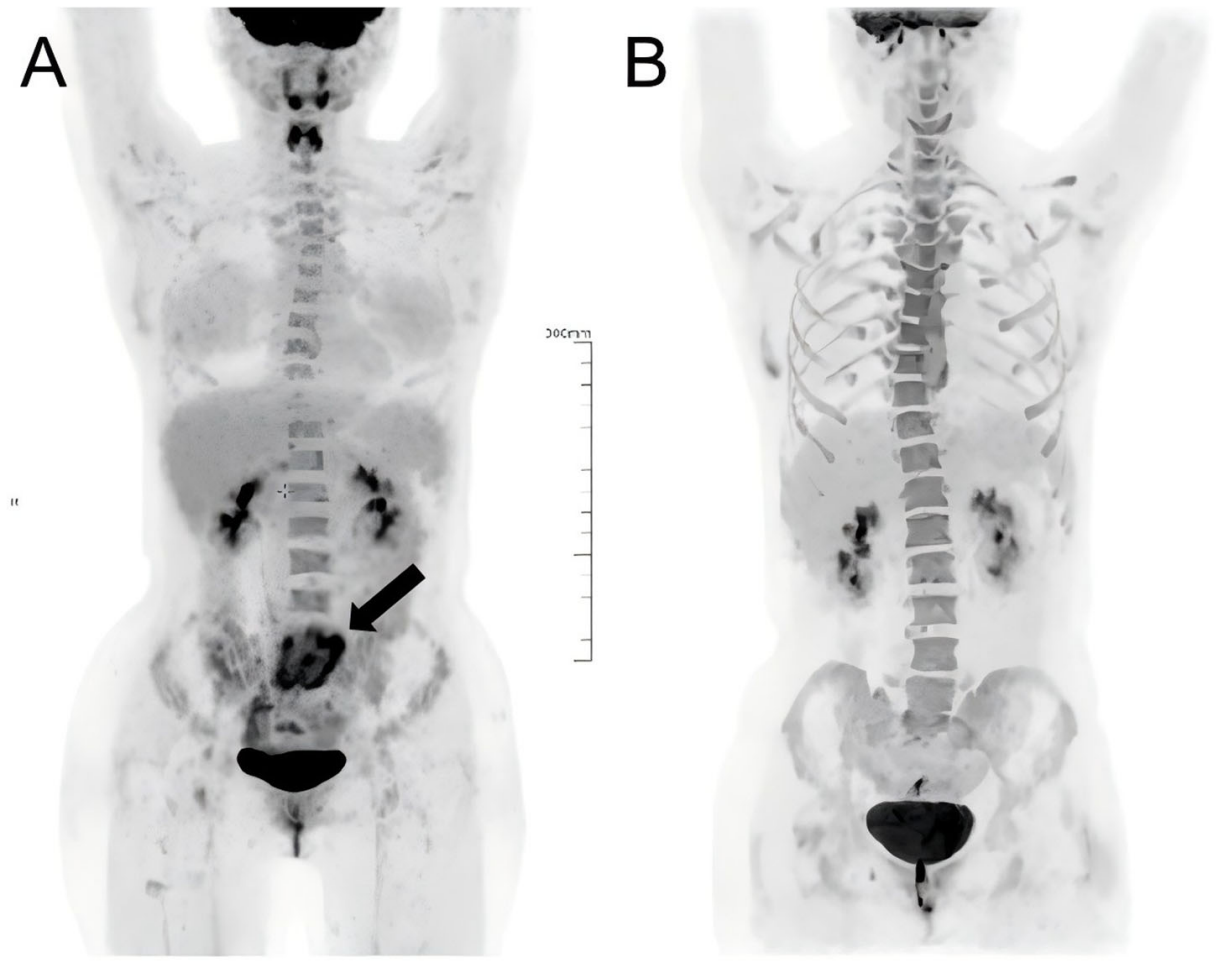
Comparison of PET/CT scans conducted (A) pre- and (B) post-treatment showed a high-uptake lesion in the patient’s abdomen (marked by the black arrow) prior to treatment, which resolved following treatment.
